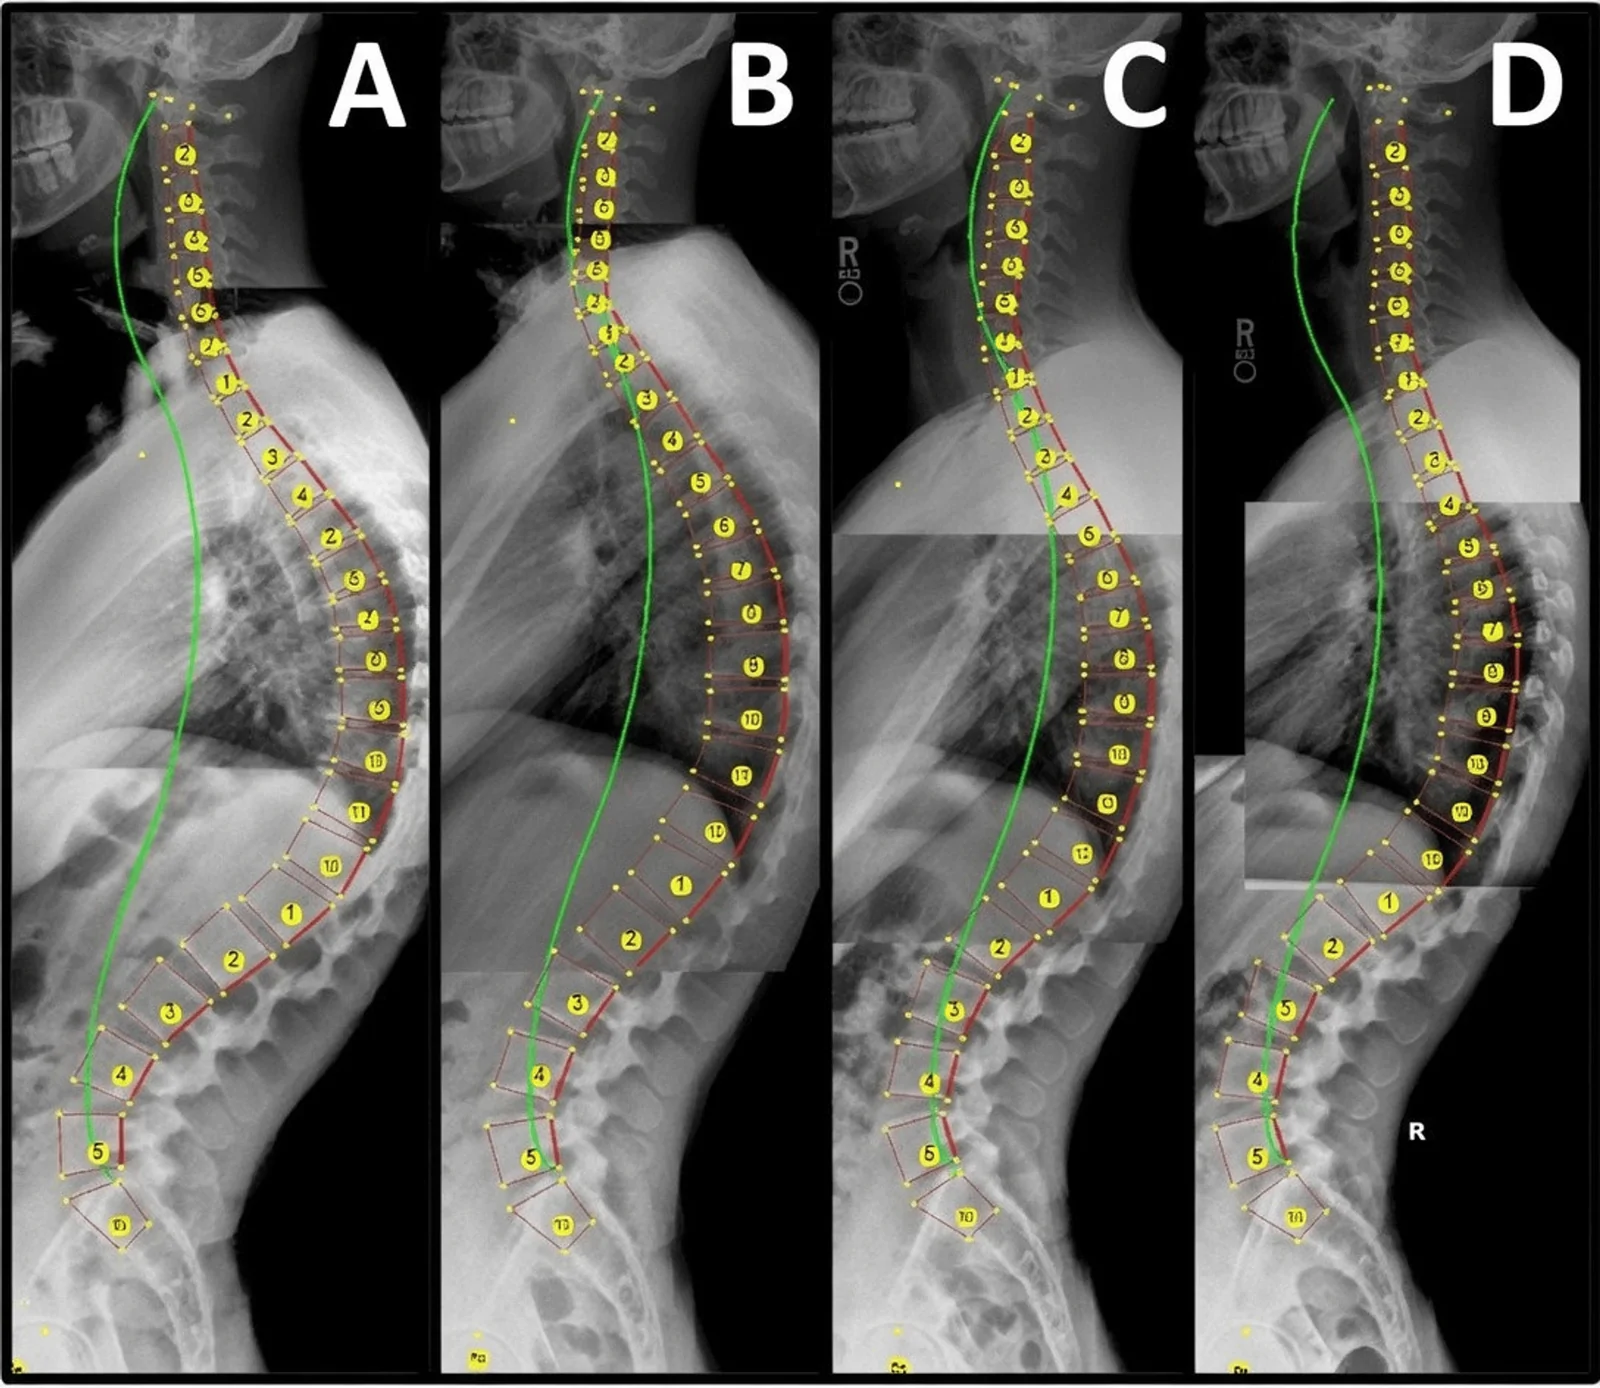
Full spine sagittal spine radiographs before, after and long-term follow-up with lines of mensuration. A) Pre-Treatment, B) Re-Exam, C) Post-Treatment, and D) Long-Term Follow-Up Sagittal Full-Spine (Stitched Sectionals) Radiographs. The green line represents a normal, ideal sagittal spinal alignment and posture. The red lines represent the C2-S1 posterior tangent lines of the patient. The black numbers in yellow circles are the numbered cervical, thoracic, lumbar, and sacral vertebrae. The yellow dots represent the marked C1 anatomical landmarks, anterior superior and inferior and posterior superior and inferior aspects of C2-S1 and hip anatomical landmarks.
